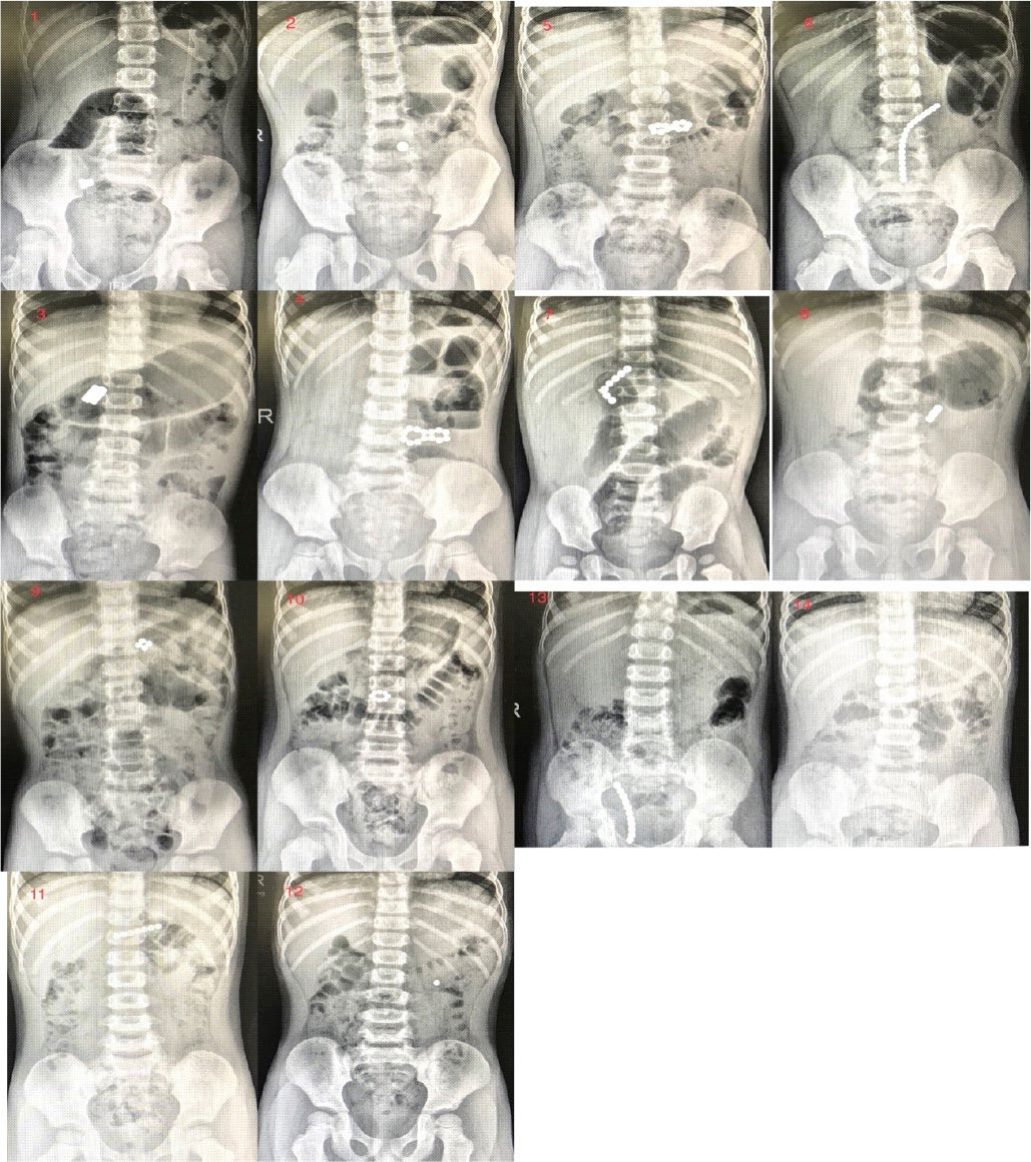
Abdominal X-rays of the 14 children with different number and different locations of the misingested magnets/magnetic beads (please refer to Tables 1, 2 in detail).
